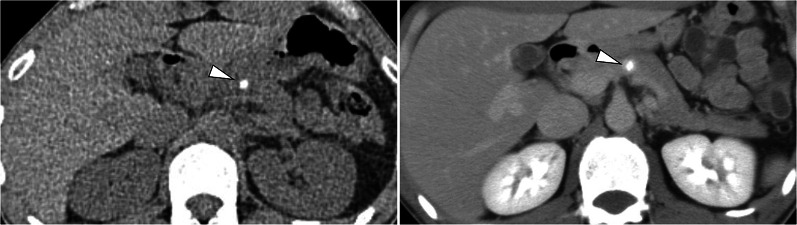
Computed tomography shows a 4-mm calcification (A) but no contrast-enhanced mass (B) in the pancreatic body
